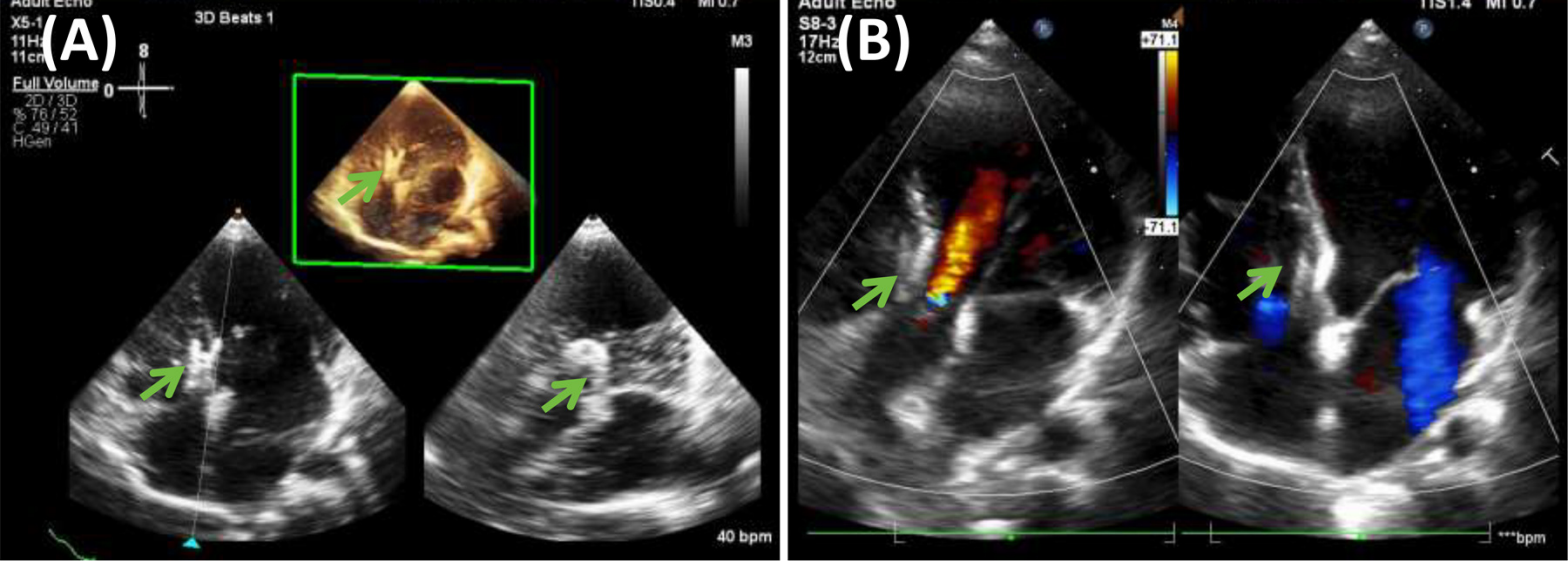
Echocardiogram findings 7 years after surgery. (A) Shows the three-dimensional image of the left atrium and ventricle, and (B) shows the apical four-chamber and five-chamber view. The area pointed by the green arrow is the eccentric occluder.
